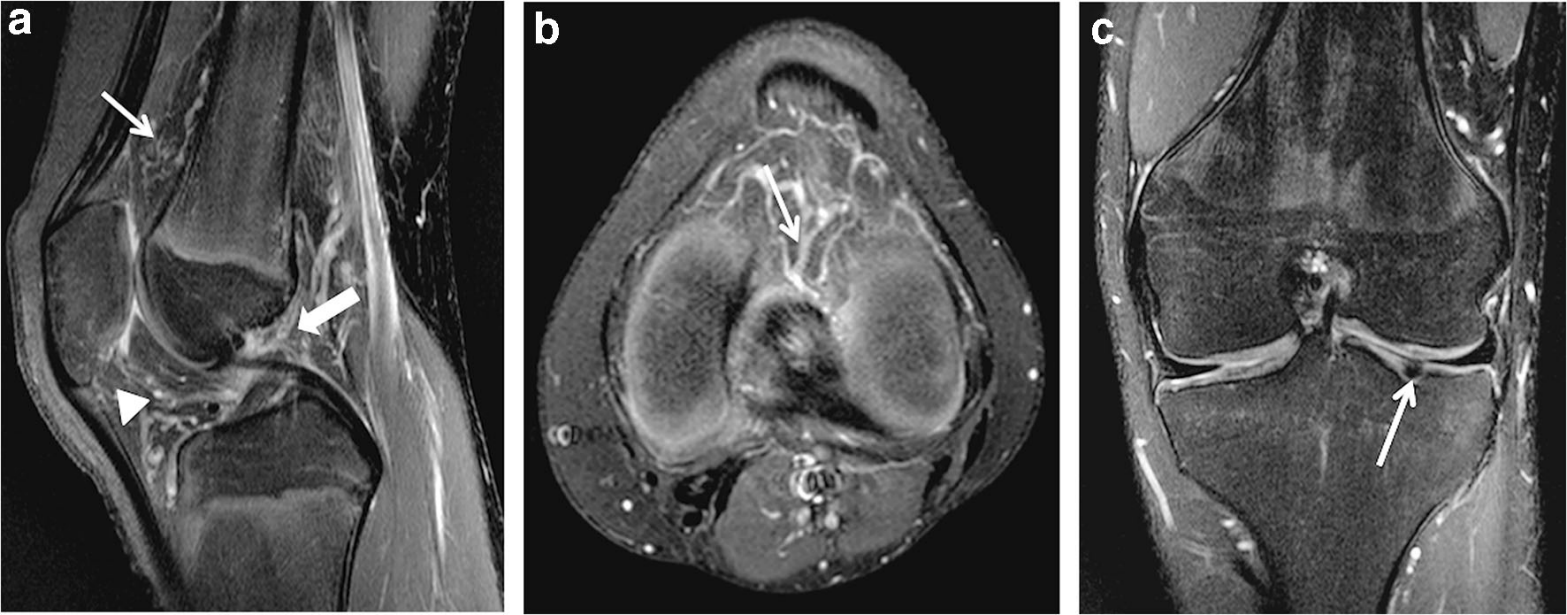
A 13-year-old boy with a diffuse intra-articular venous malformation of the knee. a A T2-weighted fat-saturated sagittal MRI shows the diffusely infiltrating phlebectatic vessels affecting the suprapatellar fat pad (*thin arrow*), infrapatellar fat pad (*arrowhead*) and intrasynovial cavity at the level of intercondylar space (*thick arrow*). b An axial T2-weighted fat-saturated MRI at the level of the intercondylar space demonstrates the pathological veins invading the intercondylar space and thus intrasynovial cavity (*arrow*). c A T2-weighted fat-saturated coronal MRI demonstrates thinning of cartilages and a full-thickness cartilage defect (*arrow*) of the same knee developed as a result of recurrent intrasynovial hemorrhages
